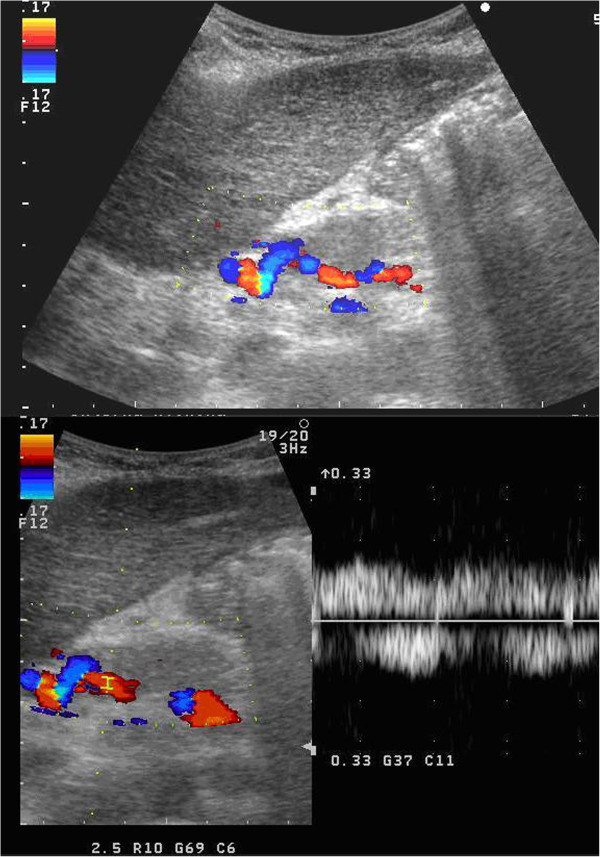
Abdominal Doppler ultrasound 2 years after right hepatectomy with reconstruction of portal vein showing normal liver parenchyma and good graft patency.
